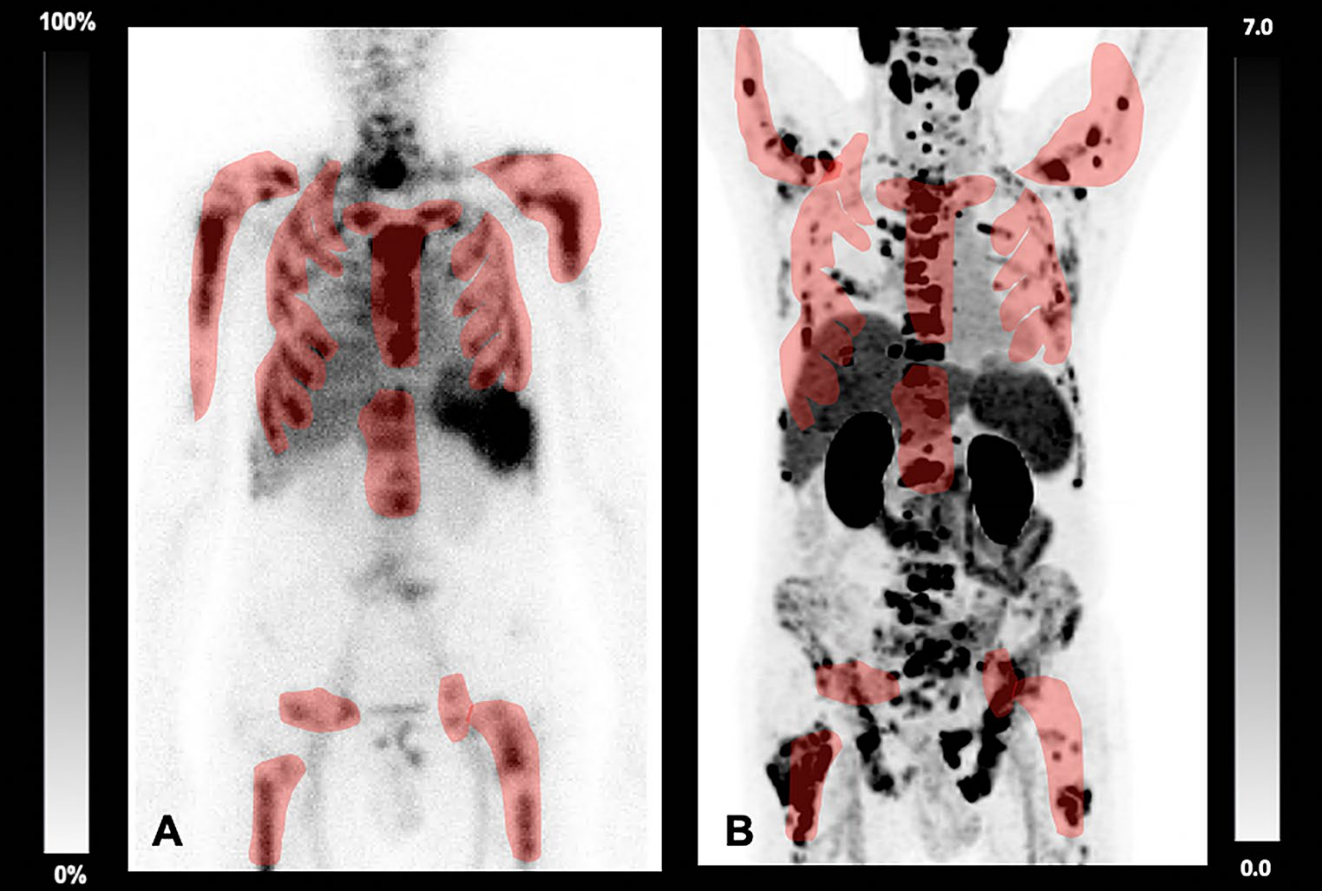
80-year-old-patient prior to PSMA-RLT undergoing A) [^99m^Tc]Tc-antigranulocyte scintigraphy and B) [^18^F]F-PSMA-PET/CT presenting with no relevant spatial overlap between viable bone marrow and osseous metastases, as demonstrated in red
